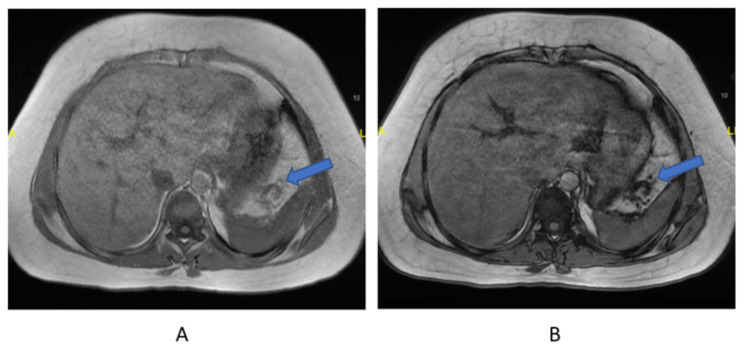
Images A and B are axial inphase and outphase Lava Flex MRI of the abdomen showing early hepatic fibrosis with diffuse moderate to severe hepatic steatosis and increased intensity of the splenic vein with multiple collaterals likely to be chronically thrombosed (blue arrows).
